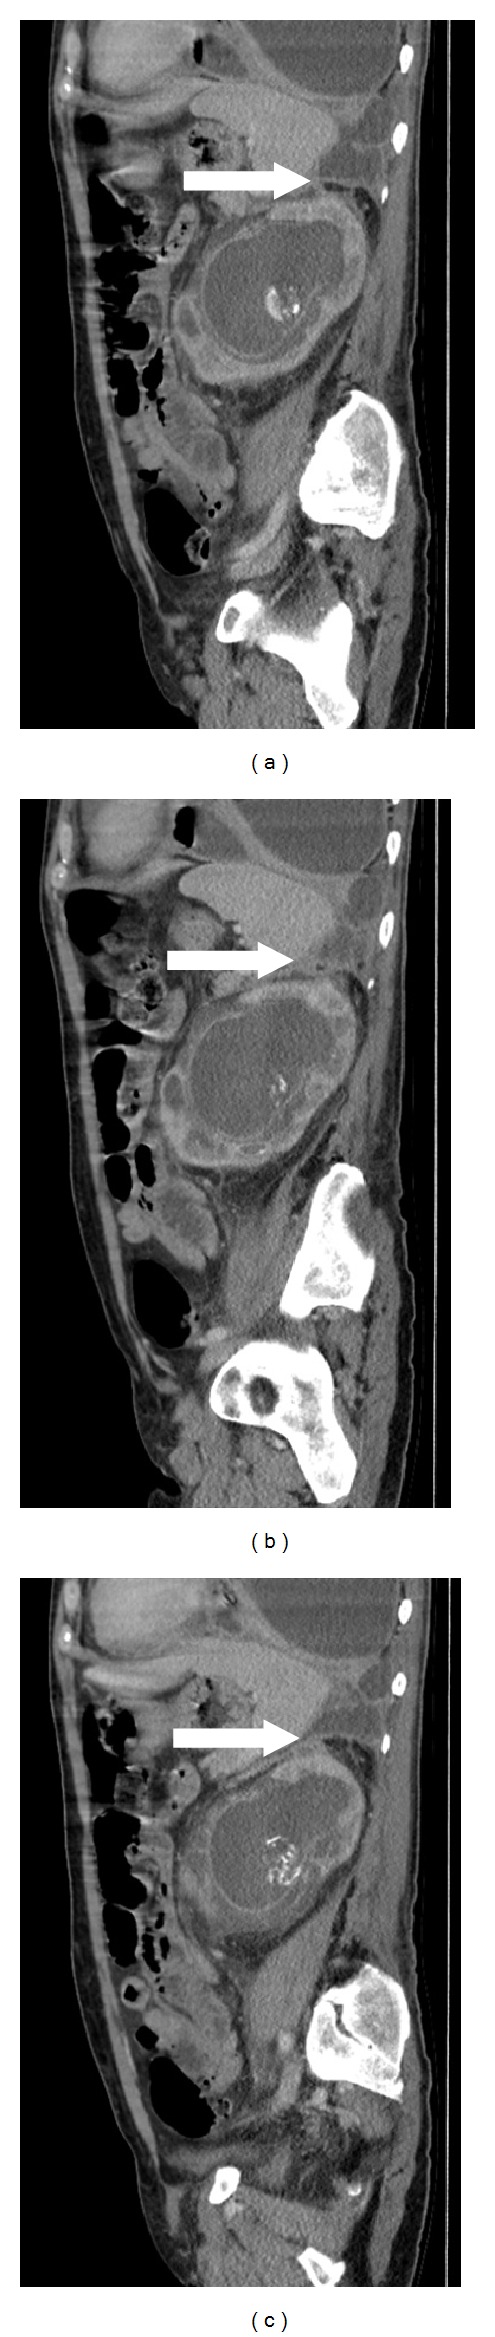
Sagittal CT images showing fistula between left kidney and pleural cavity (white arrow).
